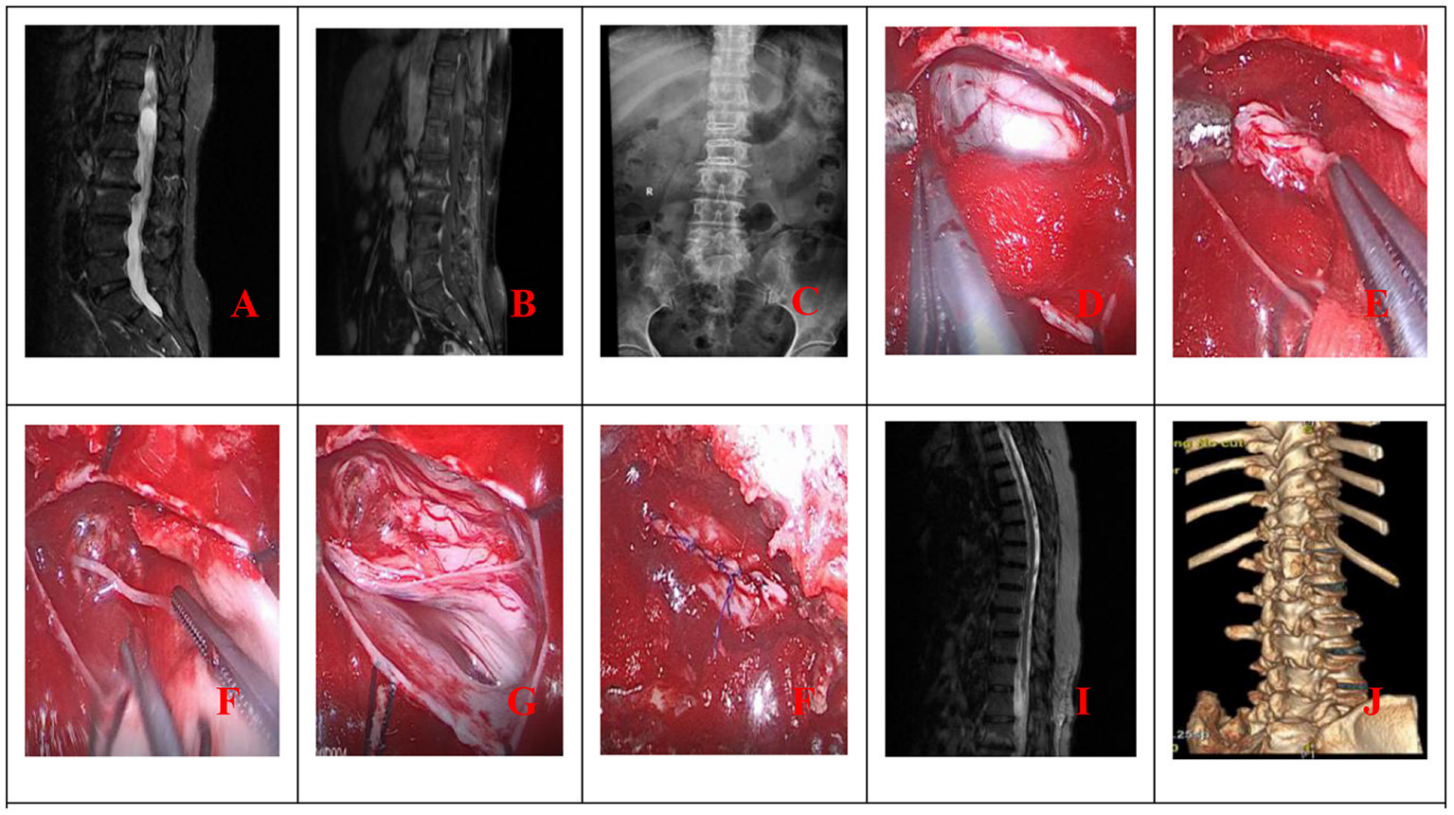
(A) Preoperative MRI; (B) Preoperative MRI enhancement; (C) Position the cone preoperatively; (D) Open the dura mater under endoscope; (E) Separate and pull out the tumor under endoscope; (F) Cut off the tumor-bearing nerve after endoscopic electrocoagulation and remove all the tumor; (G) Endoscope demonstrated good nerve root protection after tumor resection; (H) Continuous suture of spinal dura under endoscope; (I) Postoperative MRI revealed total tumor resection (J). Postoperative 3D CT reconstruction.
